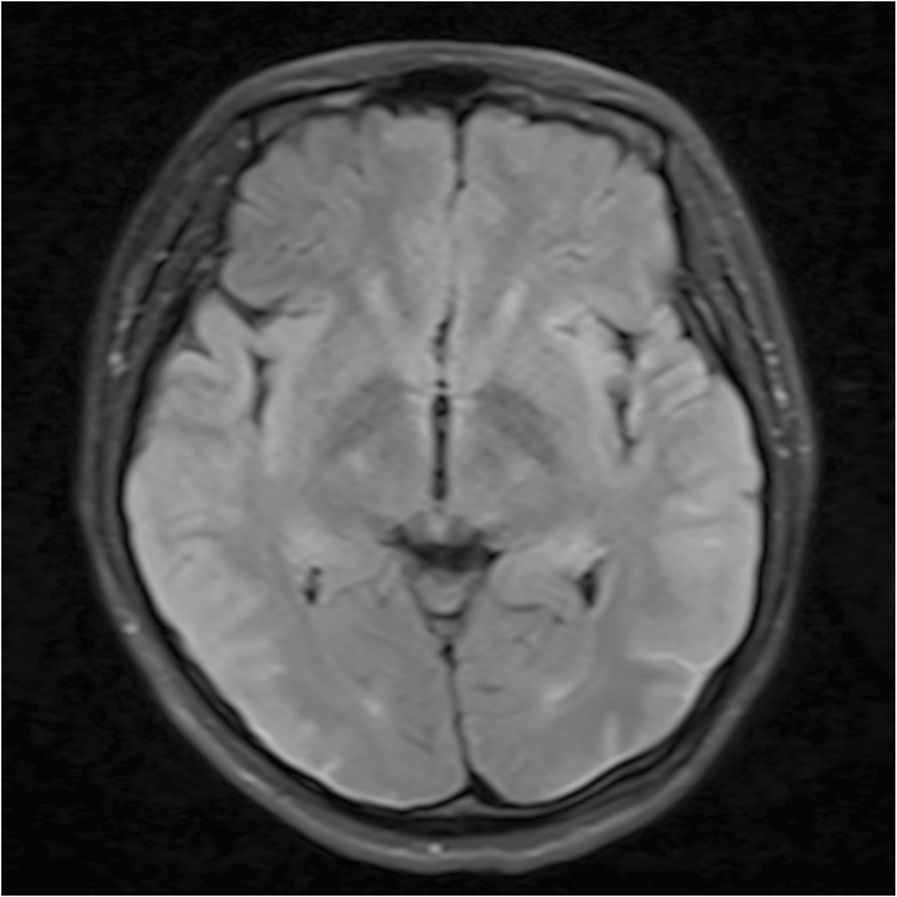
T2 fluid-attenuated inversion recovery (FLAIR) axial views showing obvious hyperintense signals and mild swilling in bilateral parietal-occipital lobe, temporal lobe and insular cortex
